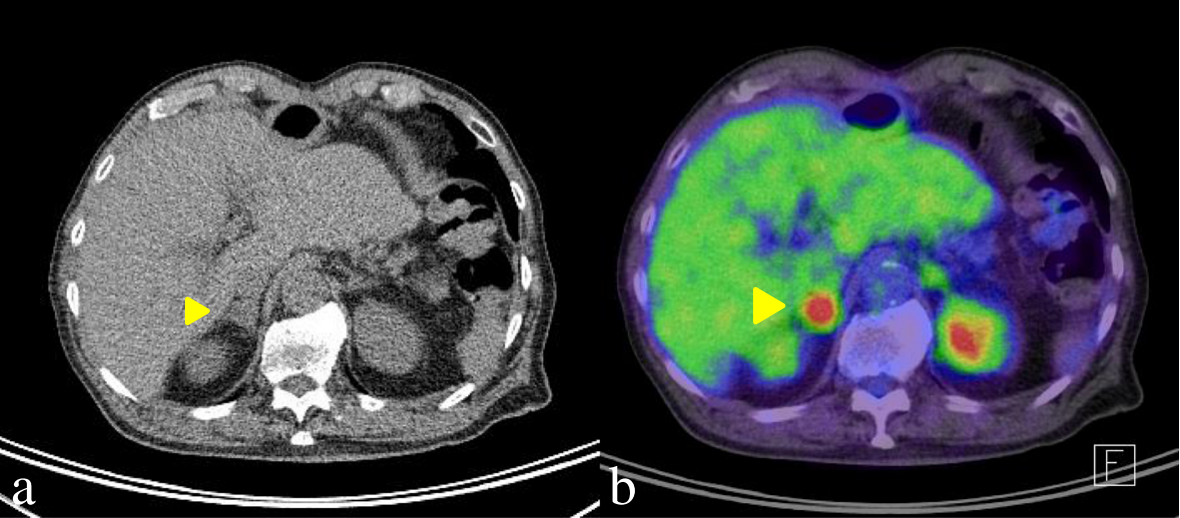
Images from CT and FDG-PET/CT. a A mass with a 2-cm diameter in the right adrenal gland. b PET/CT shows high accumulation of FDG (standardized uptake value max, 7.67) in the right adrenal mass. Abbreviations: *CT* computed tomography, *PET* positron emission tomography, *FDG* 8F-fluorodeoxyglucose
